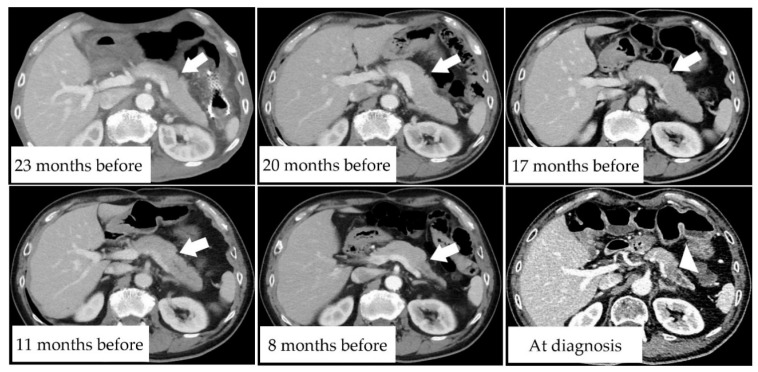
A man in his early 70s with pancreatic tail ductal adenocarcinoma (PDAC). Pre-diagnostic CT scans at 23, 20, 17, 11, and 8 months before PDAC diagnosis showing temporal changes in the pancreatic tail. White arrows indicate focal pancreatic atrophy, and the white arrowhead indicates invasive pancreatic ductal adenocarcinoma.
